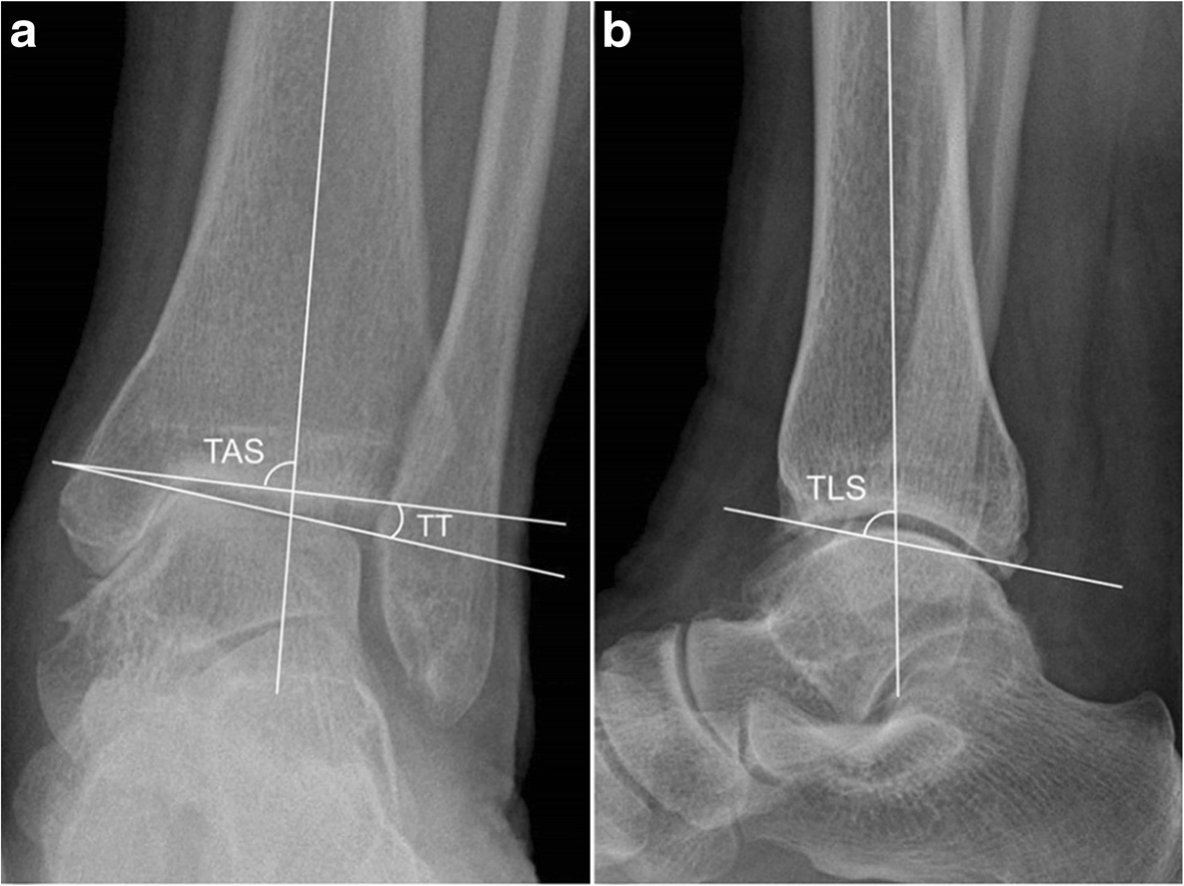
Weightbearing ankle radiographs showing the radiographic parameters used to measure alignment in ankles with varus ankle osteoarthritis. a Anteroposterior view showing measurement of the tibial-ankle surface angle (TAS) and the talar tilt (TT). b Lateral view showing measurement of the tibial-lateral surface angle (TLS)
